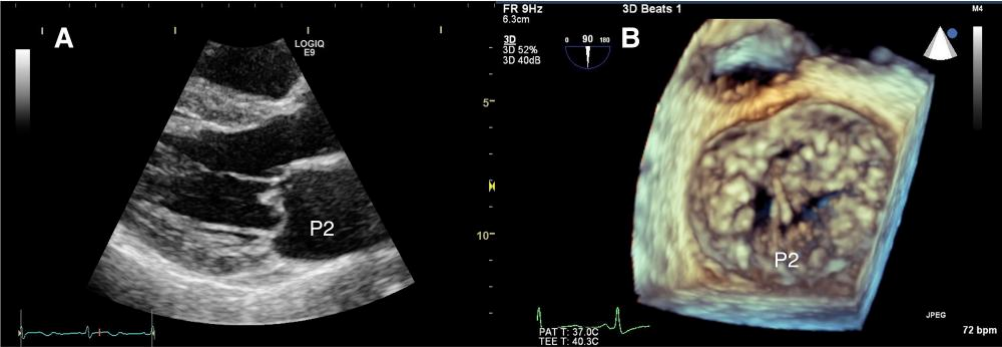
Preoperative echocardiography before initial surgical repair. (*A*) Parasternal long-axis view: P2 prolapse. (*B*) 3D transoesophageal echocardiography: P2 prolapse with chordal rupture.
